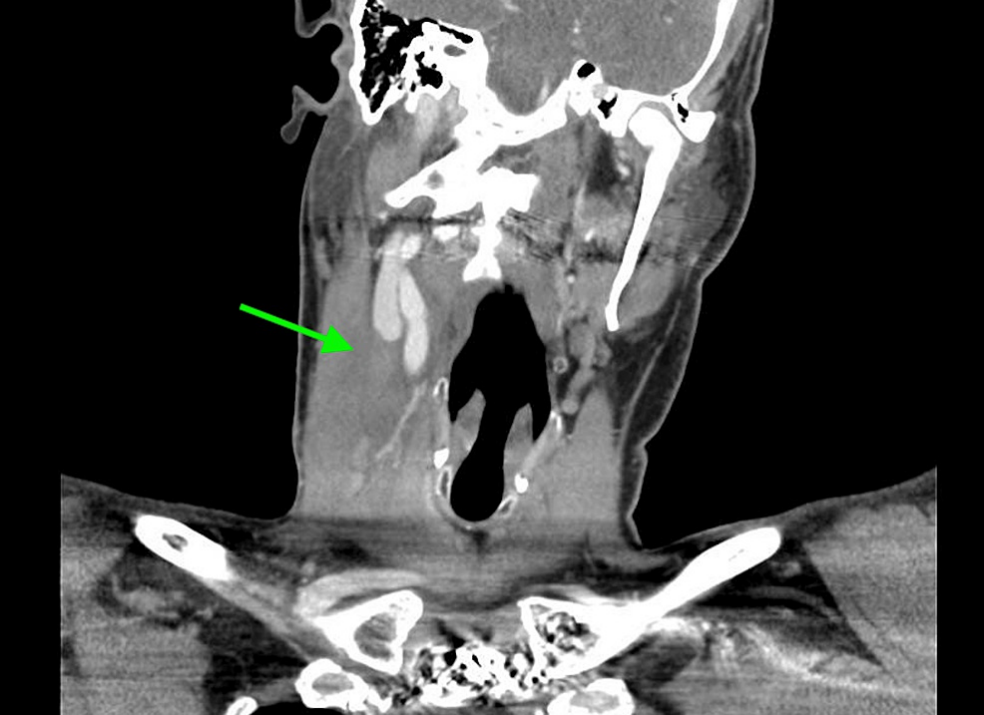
Coronal section obtained from CT of the neck with intravenous contrast demonstrates a sudden loss of contrast opacification in the right internal jugular vein, accompanied by surrounding stranding and edema, suggesting thrombophlebitis with no discernible fluid collection amenable to drainage (green arrow).
